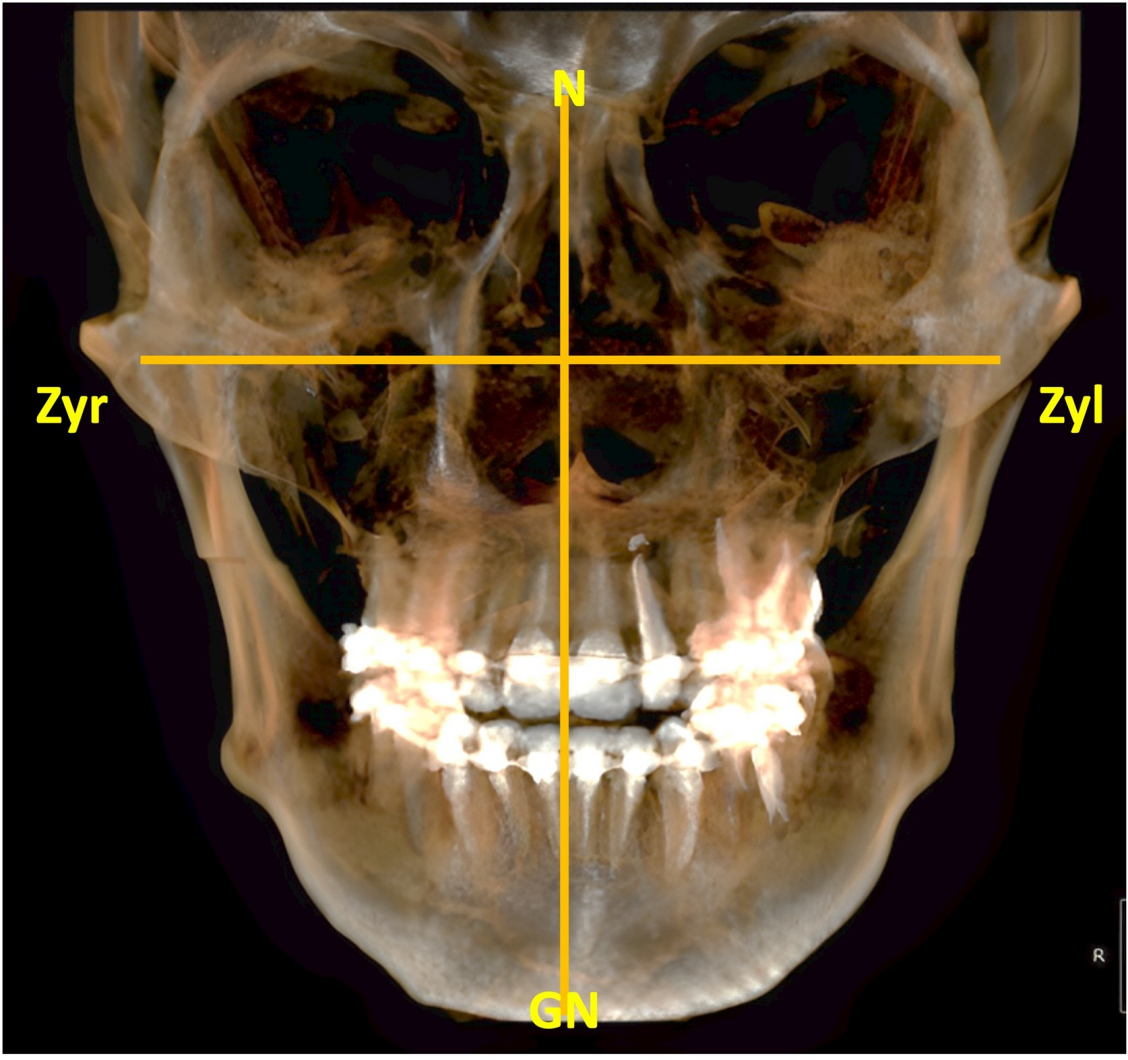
Panfacial CBCT image to calculate facial index.
